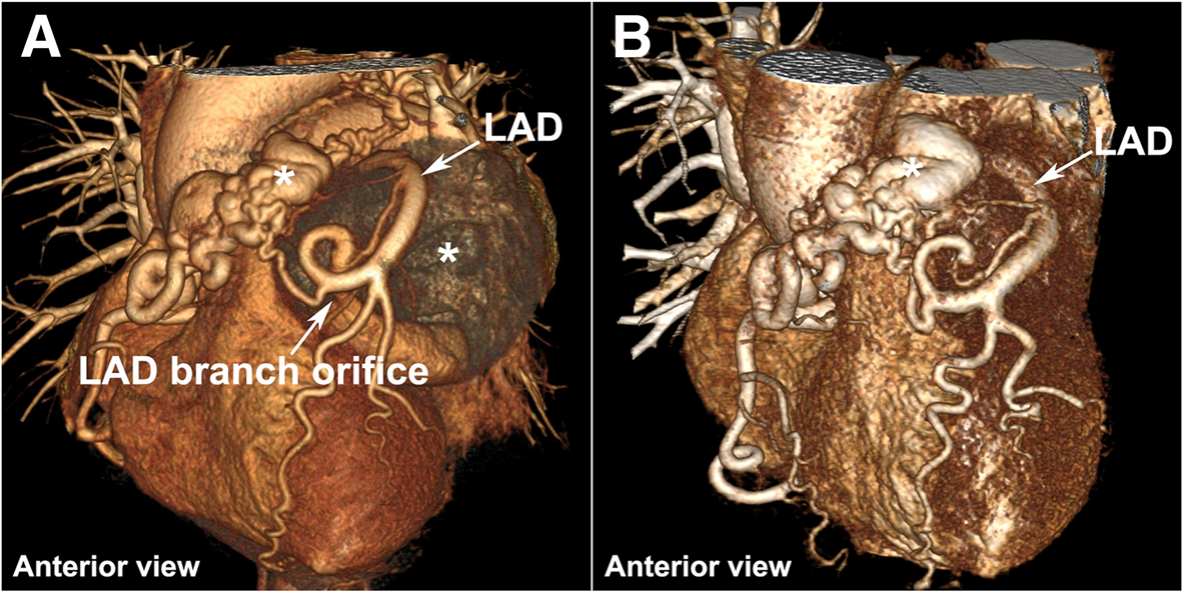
Anterior views of perioperative three-dimensional volume-rendered CTA. a Preoperative; b Postoperative. LAD, left anterior descending coronary artery; CTA, computed tomography angiography; *, coronary artery aneurysm
